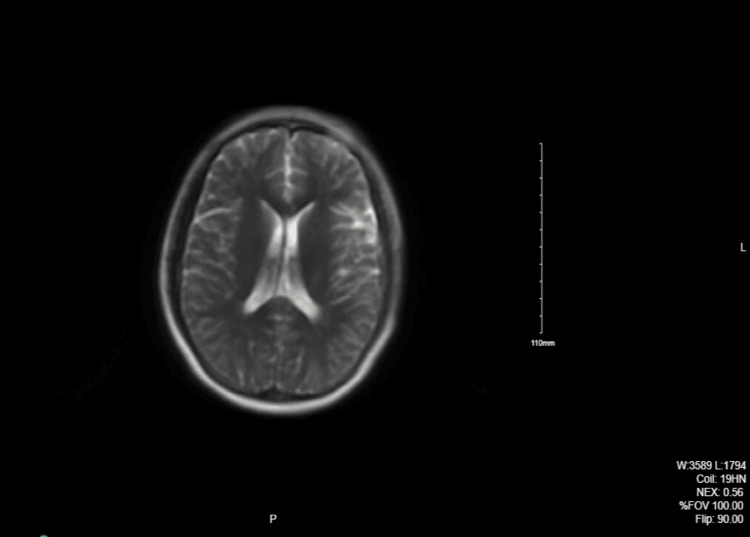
MRI of the brain with contrast: Normal study.
